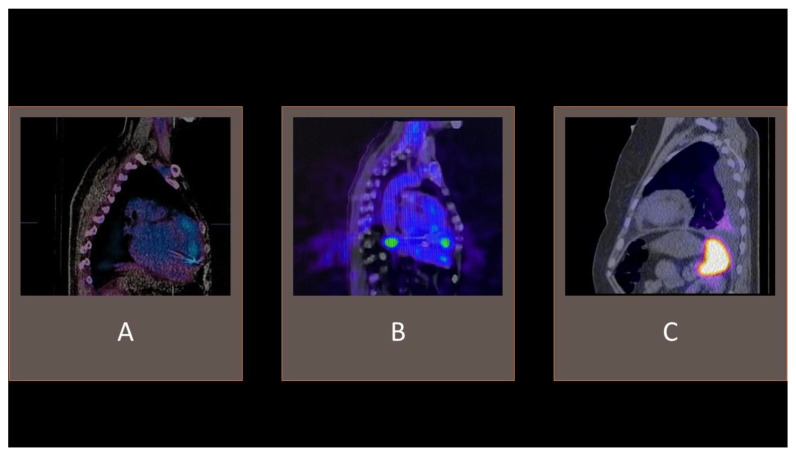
Examples of ^99m^Tc-HMPAO-SPECT/CT. (A) tracer uptake consistent with cardiac device-related infective endocarditis with an accumulation of radiolabeled leucocytes in the vicinity of an implanted electrode. (B) visible tracer uptake in the vicinity of an implanted left ventricle assist device. (C) accumulation of radiolabeled leucocytes in the pleural cavity effusion, without pathological intracardiac uptake.
